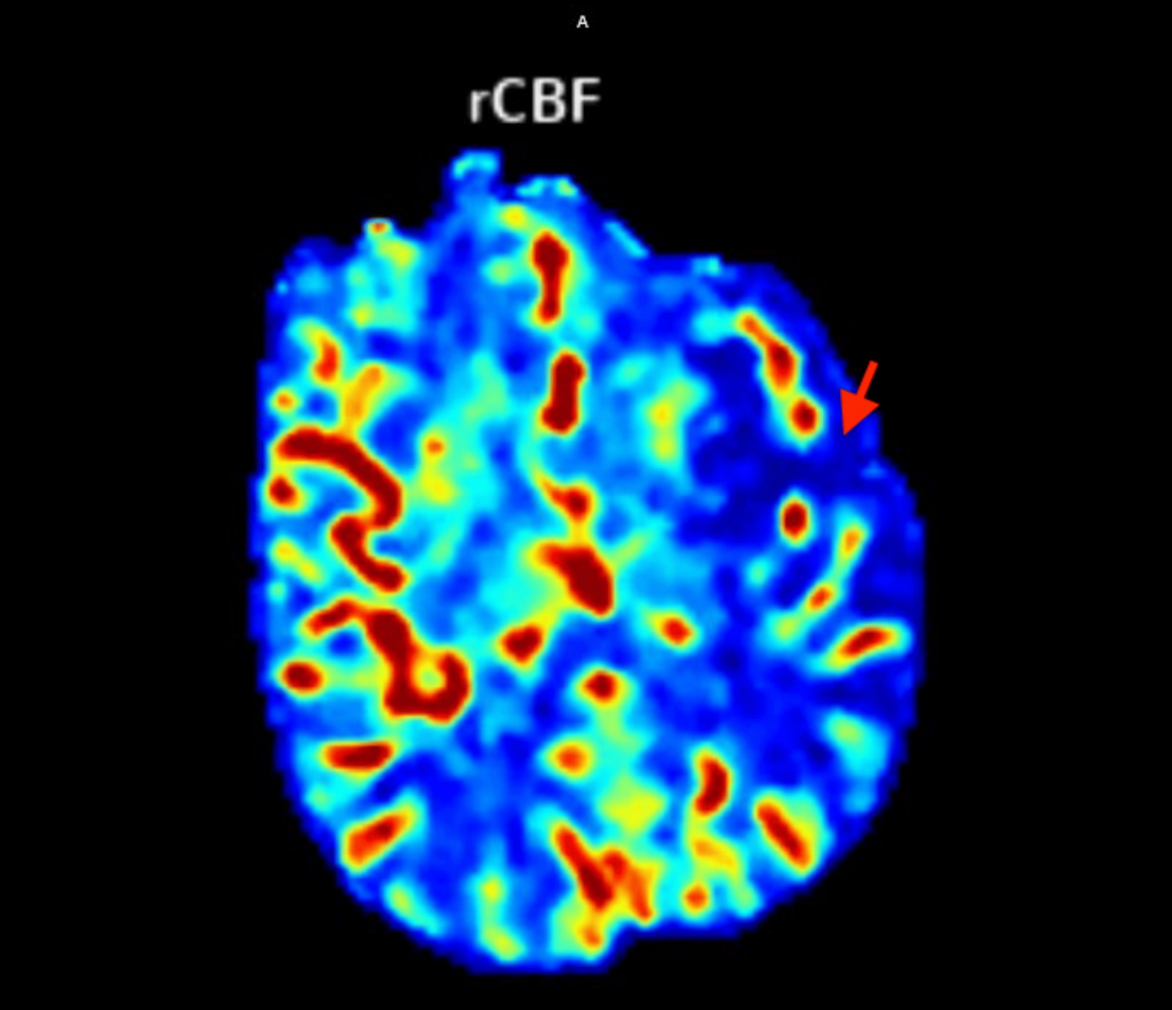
CT cerebral perfusion scan showing a large area of ischemia throughout the middle cerebral artery distribution (red arrow) rCBF - regional cerebral blood flow
